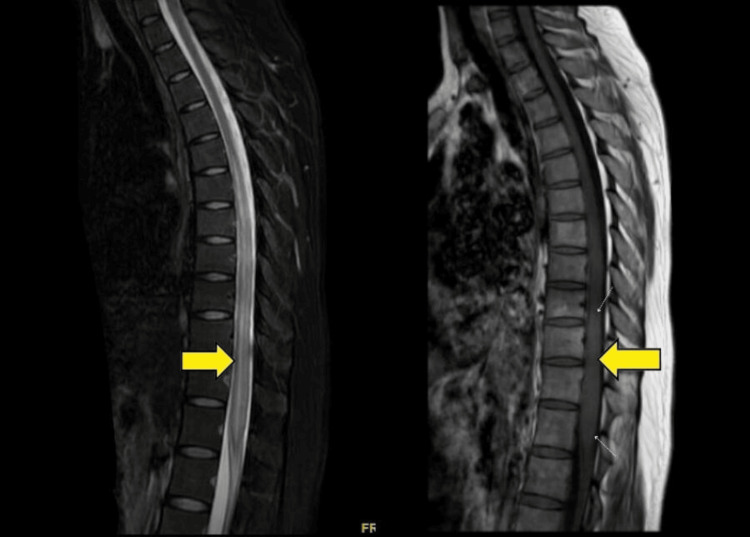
Contrast-enhanced MRI of the dorsolumbar spine: (A) MRI T2 images suggestive of the intramedullary hyperintense lesion at the D7-D9 level (yellow arrow); (B) MRI T1 image showing a long-segment hypointense lesion at the D7-D9 level (yellow arrow). MRI, magnetic resonance imaging
